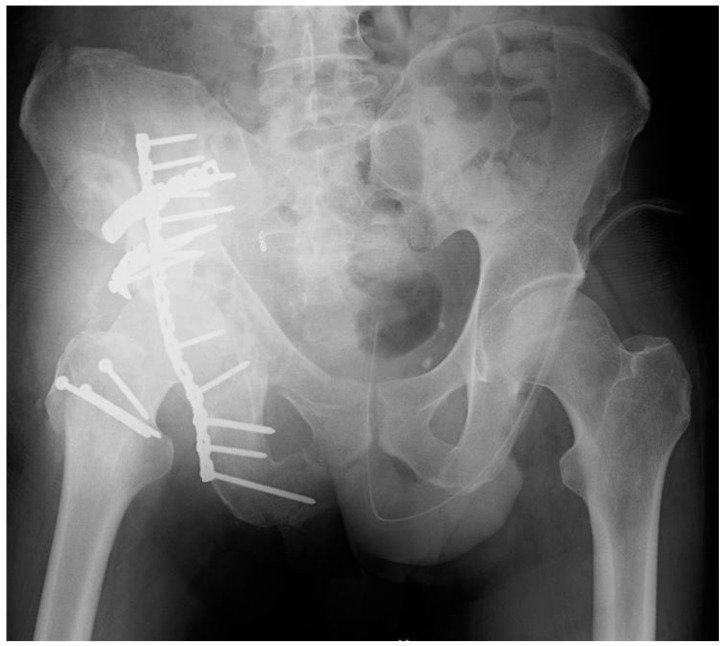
X-ray of the pelvis and hip when the patient came to our attention.
